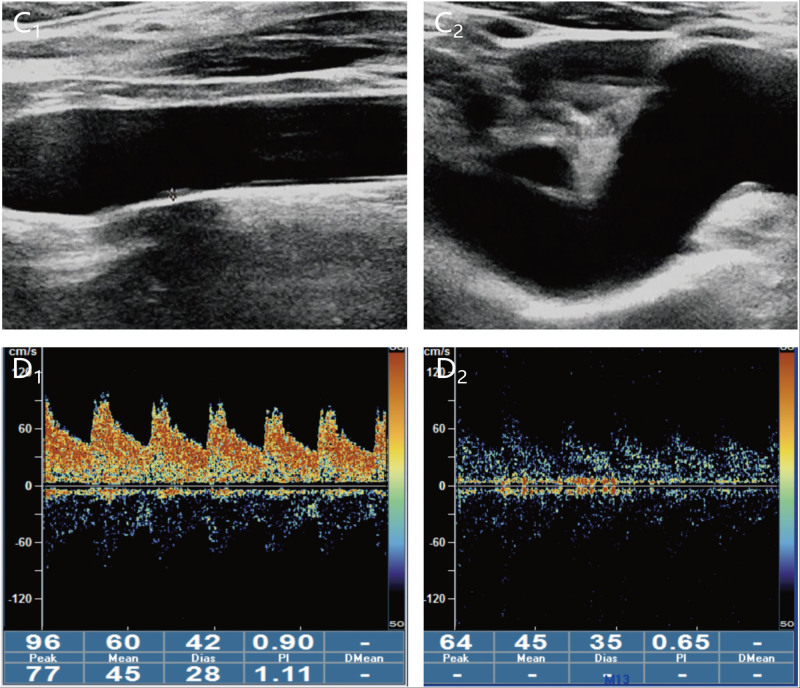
Carotid Ultrasound and Transcranial Doppler bubble Test. (C_1_ and C_2_, D_1_ and D_2_) No obvious abnormality was found in the carotid ultrasound and transcranial Doppler bubble test.
